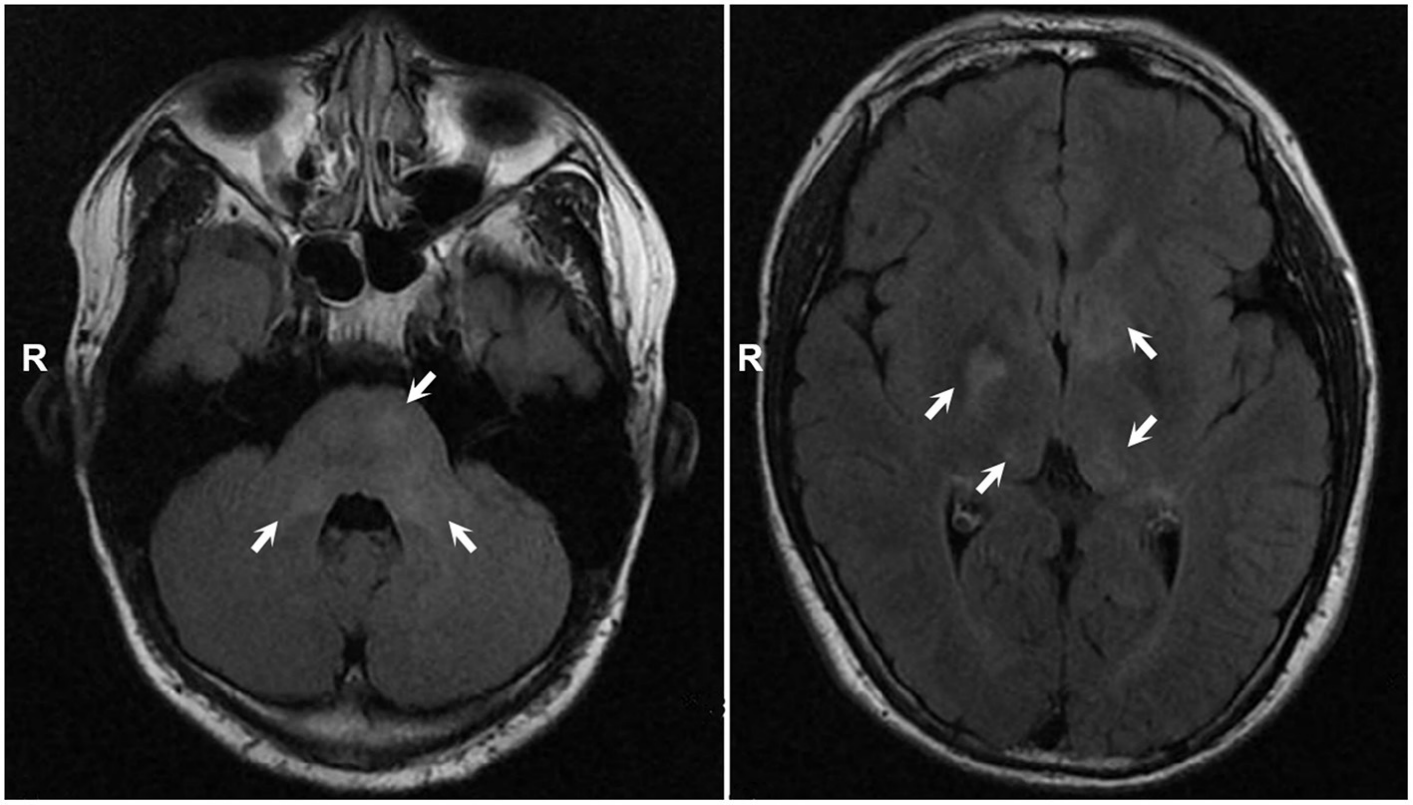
MRI showed multiple abnormal signal foci in bilateral basal ganglia, thalamus, and pons.
